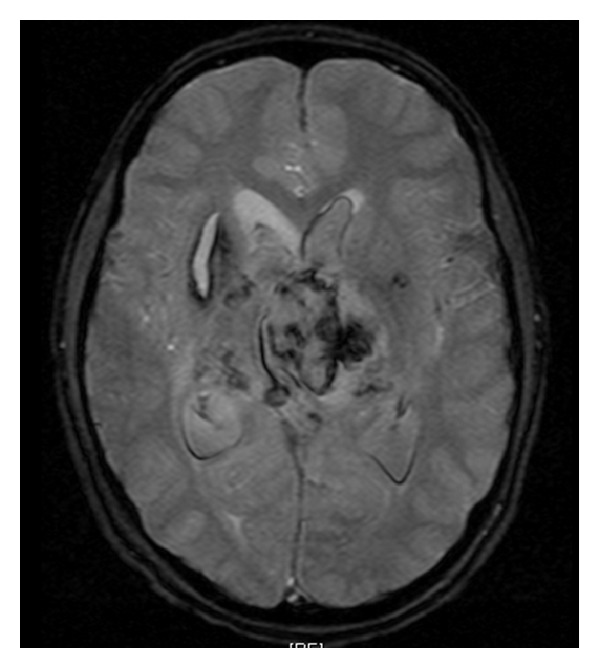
Axial GRE-heme sequence MRI 8 months after diagnosis, during presentation with status epilepticus.
